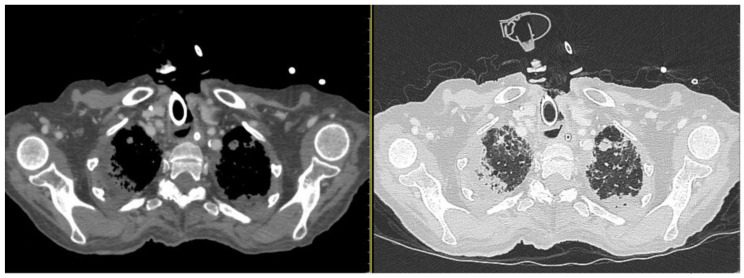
CT scan windows showing metastasis and inflammatory phenomena on both sides of the lung. (On the left, axial parenchymal lung section. On the right, axial lung window). During hospitalization, bacterial pneumonia began, worsened by secondary viral (Cytomegalovirus) and fungal pneumonia. The patient received a triple antibiotics course with an antiviral, an antifungal, and systemic and local inhalational corticosteroids. However, despite the medical therapy, the chest condition deteriorated progressively (Figure 4).
